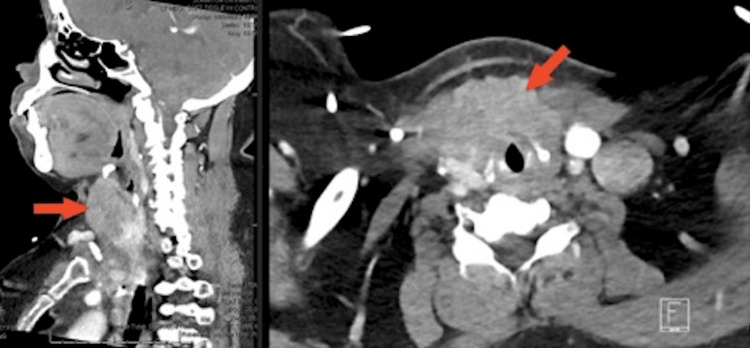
CT of the chest with contrast showing a heterogeneous thyroid lobe mass measuring 4.9 x 3.0 cm (arrows) without mass effect on the respiratory tract. CT, computed tomography
